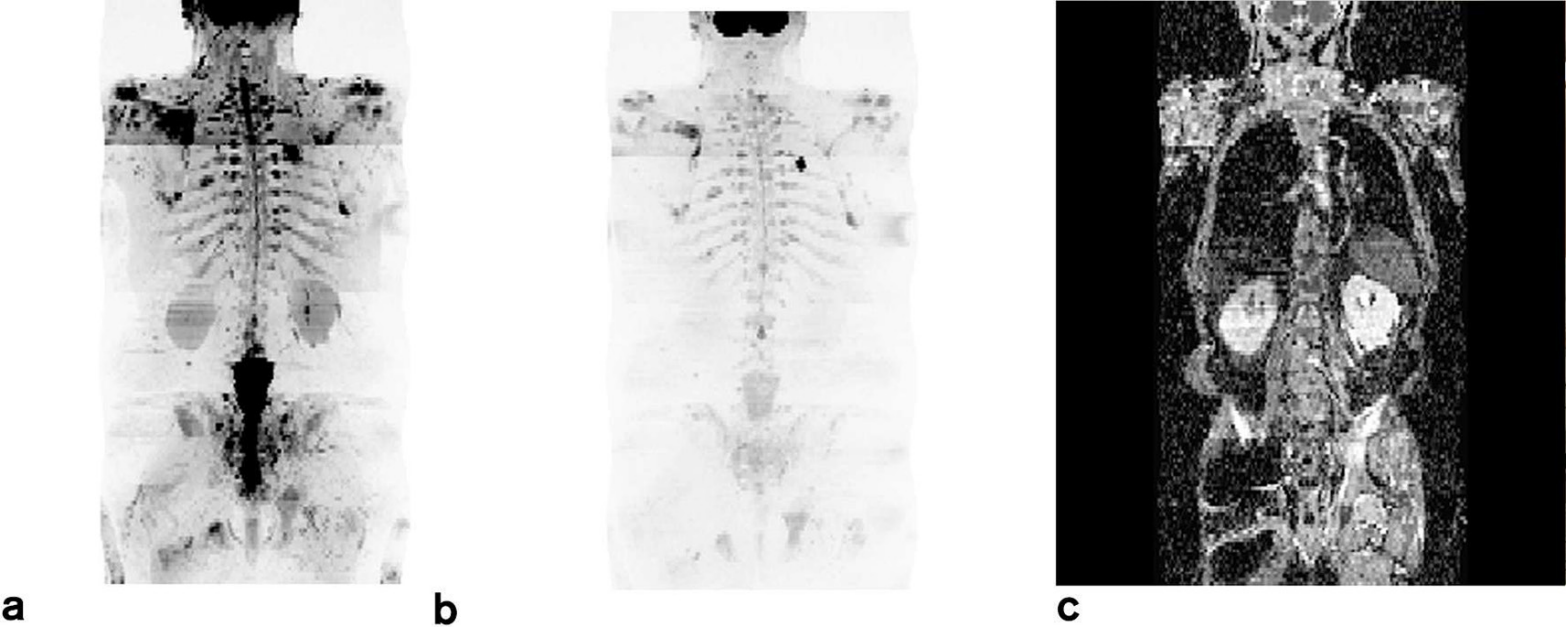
Example of a qWB-DWI data set from a patient with advanced prostate cancer (a) b = 50 s mm^–^^2^, (b) b = 900 s mm^–^^2^ and (c) corresponding ADC map calculated using a mono-exponential model. ADC, apparent diffusion coefficient; WB-DWI, whole body diffusion-weighted MRI.
